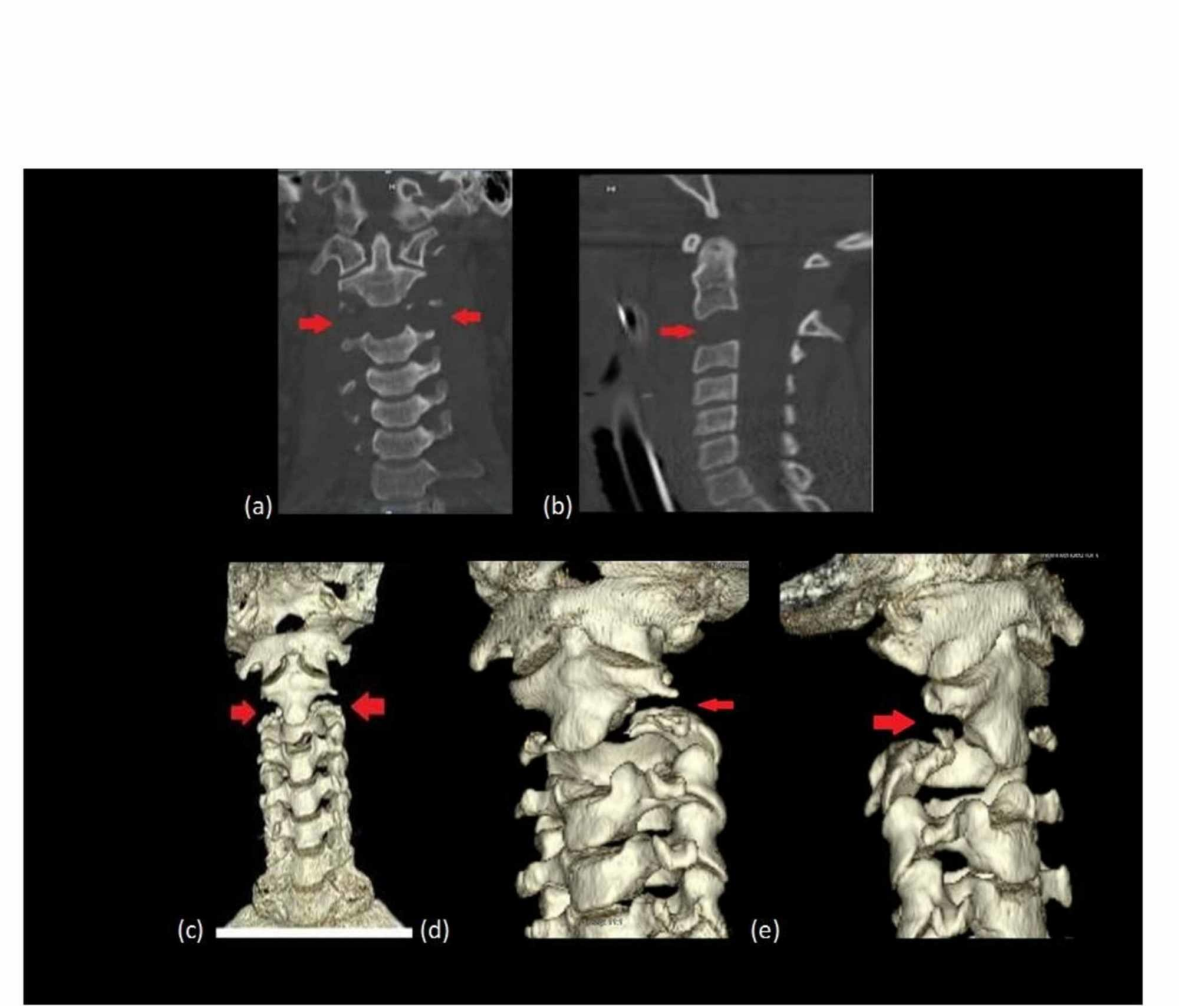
CT of the cervical spine: (a) coronal, (b) sagittal, (c) CT 3D reconstruction (d) and (e) 3D oblique view. All views with red arrows show cranial caudal dislocation of C2 on C3.
